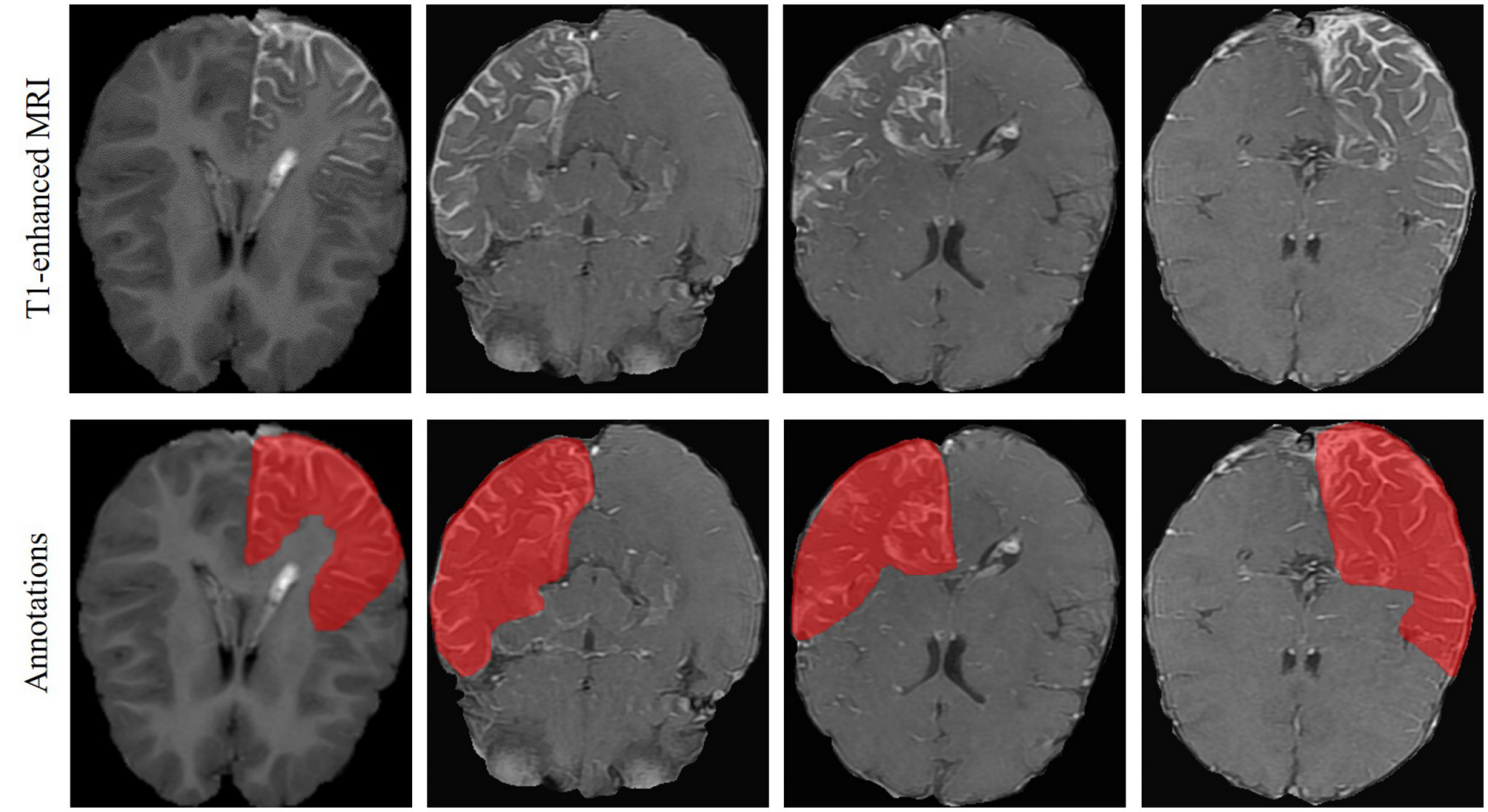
LA in T1-enhanced brain MRI of the SWS patients and the corresponding annotations.
